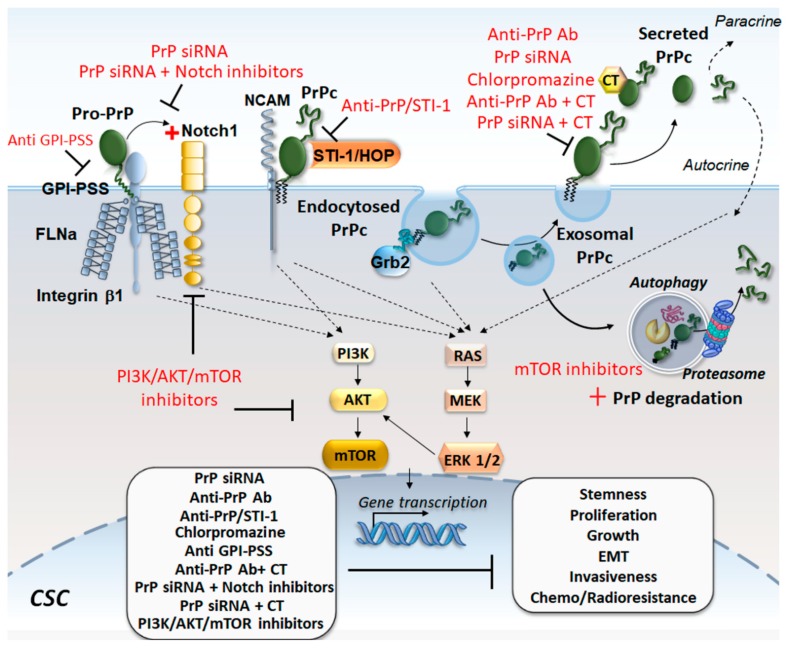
Targeting the cellular prion protein (PrPc) in cancer stem cells (CSCs). Strategies aimed at downregulating/inhibiting PrPc in CSCs include anti-PrP antibodies, PrP silencing (siRNA), administration of chlorpromazine, anti-PrP/stress-inducible protein 1 (STI-1)/Hsp70/Hsp90 organizing protein (HOP) peptides, PI3K/Akt/mTOR inhibitors, and combined strategies such as anti-PrP antibodies or PrP silencing (siRNA) with chemotherapy (CT), and PrP silencing with Notch inhibitors. These strategies counteract CSCs’ stemness, self-renewal, growth, proliferation, epithelial to mesenchymal transition (EMT), invasiveness, and resistance to radio/chemotherapy. PI3K/Akt/mTOR inhibitors also counteract PrPc accumulation by fostering its degradation through the cell-clearing pathways’ autophagy (ATG) and proteasome (red cross PrP degradation). ATG activation also downregulates Notch signaling, which is enhanced upon association with PrPc (red cross Notch1). In this way, PrP-induced pathways sustaining CSCs’ phenotype and PrP exosome release are toned down. This is key, as secreted PrP acts via autocrine and paracrine mechanisms to foster CSCs’ phenotype and can limit therapeutic drugs’ efficacy via direct sequestration of CT. Black solid lines indicate molecular steps (arrows: Stimulation; “T”-shaped lines: Inhibition); black dotted arrows indicate PrPc-related molecular steps leading to the activation of intracellular signalling pathways.
